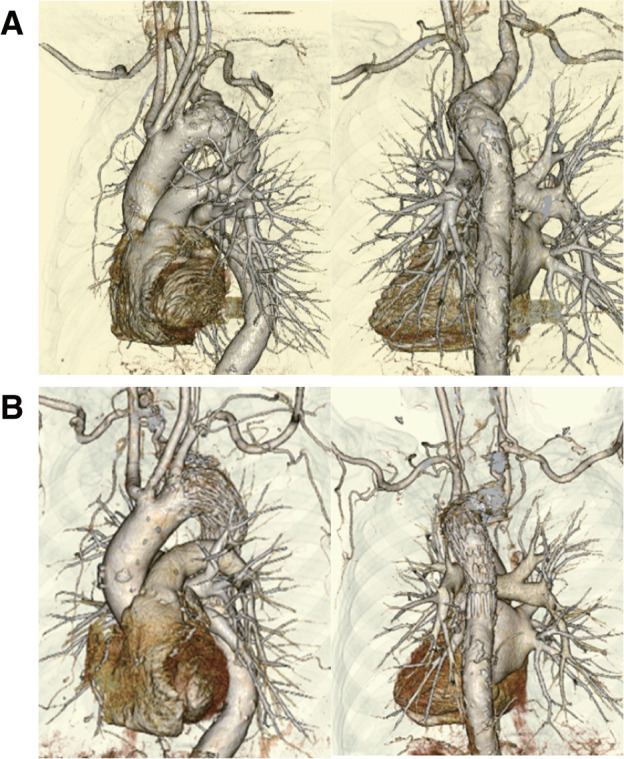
Representative 3D-CTA images of a patient with Kommerell’s diverticulum. (A) Preoperative 3D-CTA. (B) Postoperative 3D-CTA. 3D: 3-dimensional; CTA: computed tomography angiography
